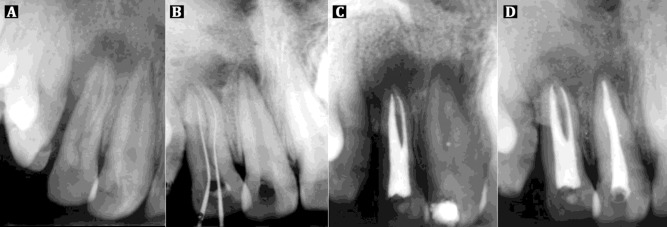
A) Preoperative periapical radiograph; B) Working length periapical radiograph; C) Post obturation periapical radiograph; D) Follow-up radiograph after 1 year
